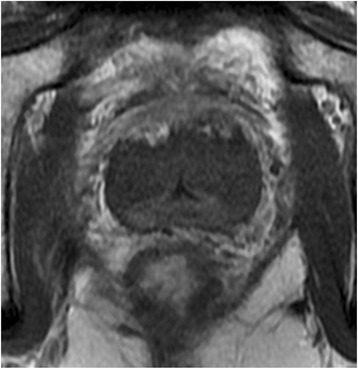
T1 W tranvers MRI, 1 min post Gadolinium contrast agent. The lack of contrast agent uptake is indicative of tissue coagulation
